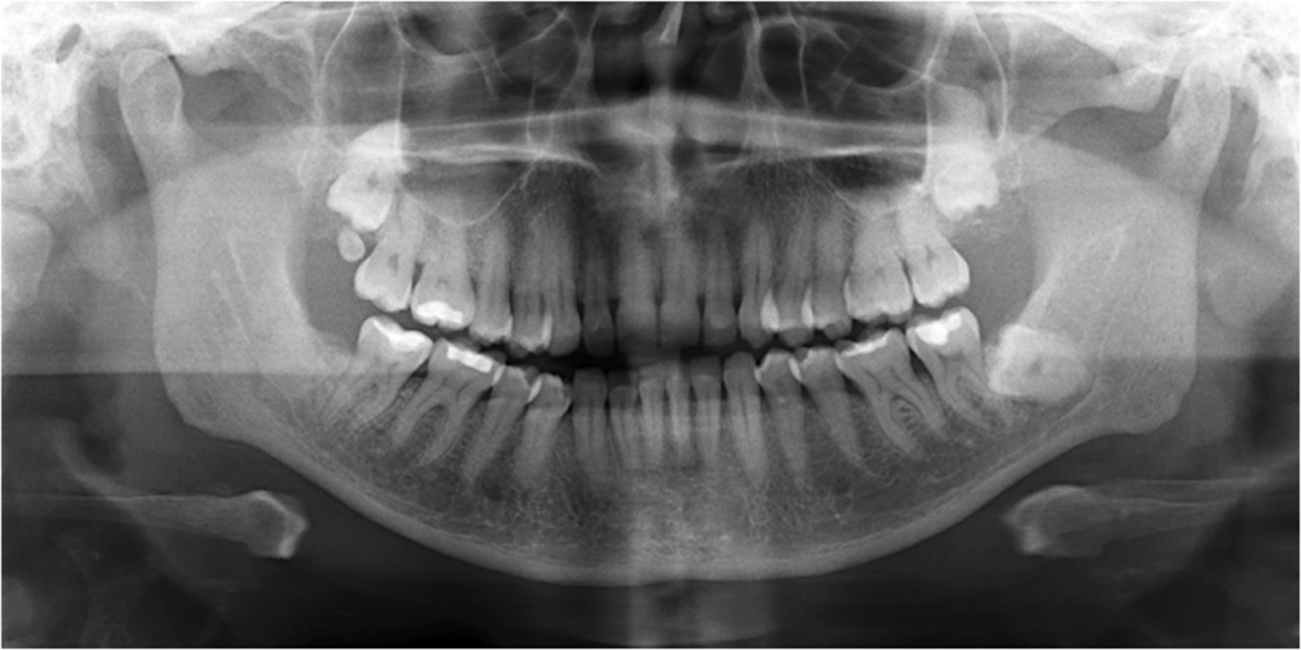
Panoramic radiograph depicting a patient presenting with numbness of the right lip and chin. The removal of the lower wisdom tooth on the corresponding side occurred one year ago. The radiograph clearly displays the lower border of the nerve canal of the inferior alveolar nerve, with a somewhat blurred upper border. Notably, a tooth-shaped dense region is evident, overlapping the nerve canal, raising concerns about a potential injury to the inferior alveolar nerve
